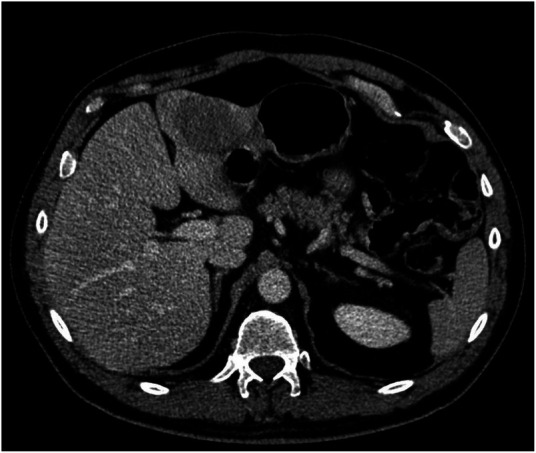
Abdominal CT scan showing a 5 cm lesion on segment 3.
